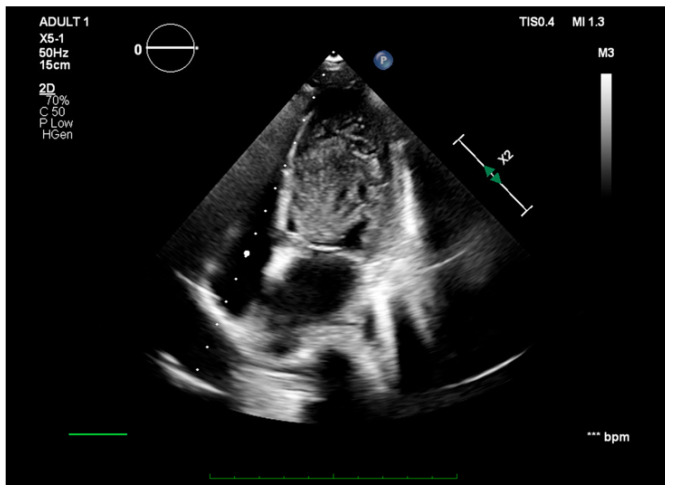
Apical four-chamber view showing a massive left ventricular tumor attached to the apical region and the interventricular septum.
